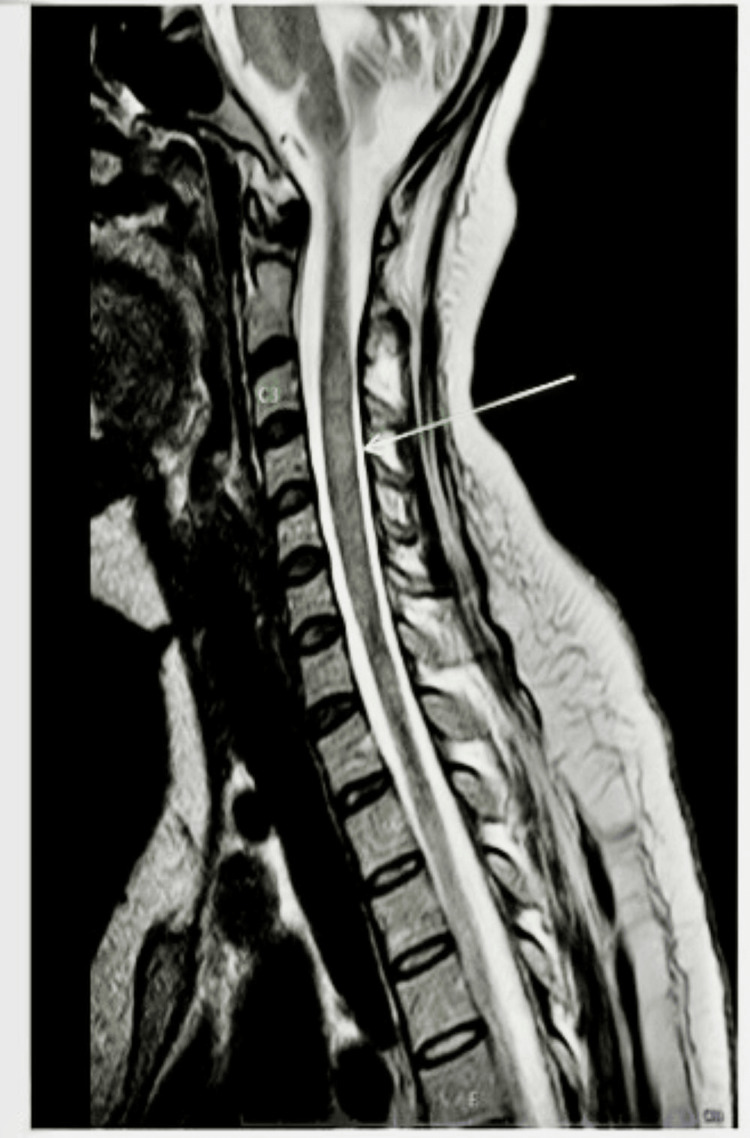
MRI spine (T2-weighted sagittal section) shows a hyperintense longitudinally extensive lesion extending from C3 to T2 Arrow indicates the area of spinal cord involvement
